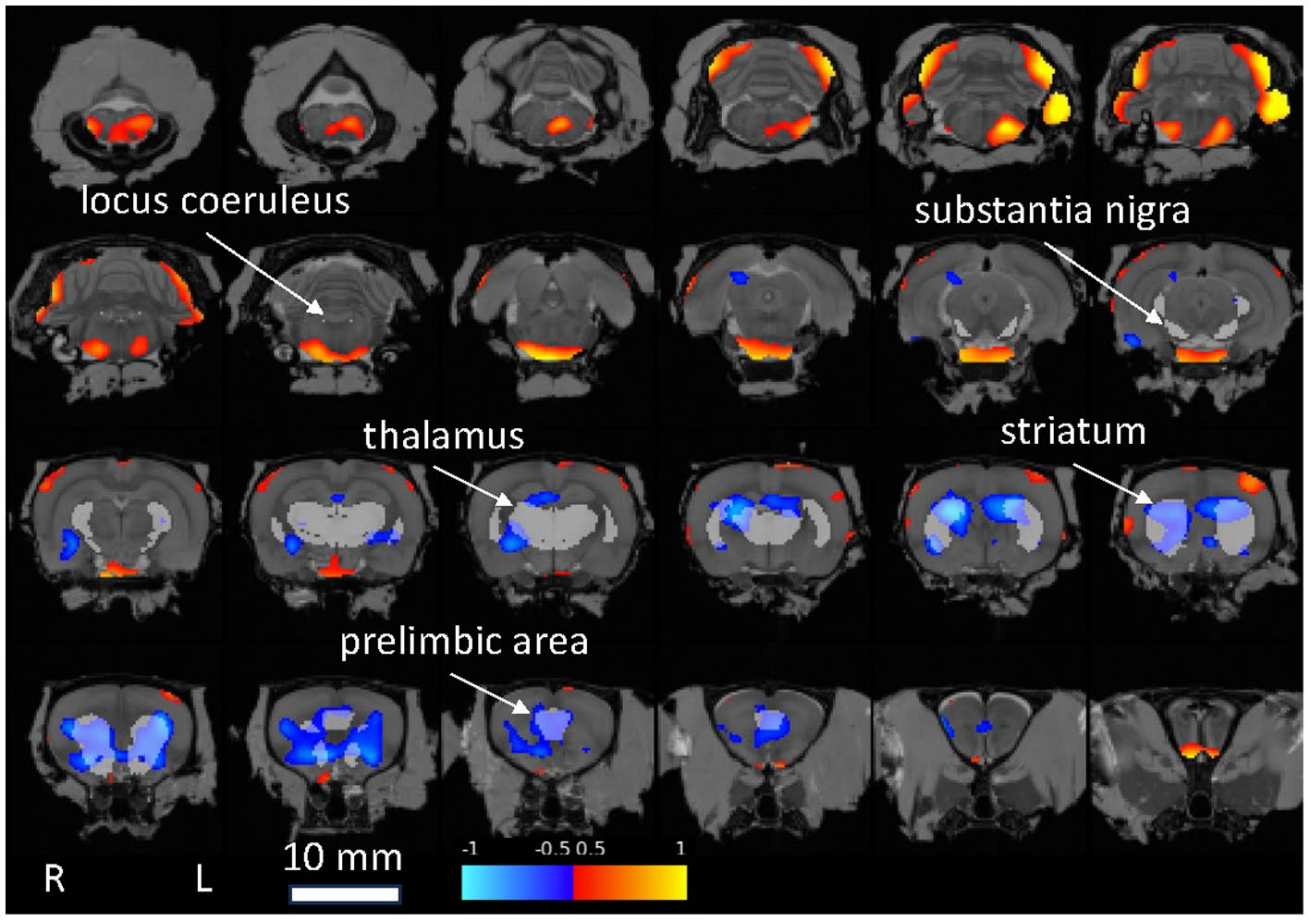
Statistical map showing *Pink1−/−* whole-brain normalized FDG uptake images compared against wildtype images (two-sample *t*-test). Red: *Pink1−/−* > wildtype; blue: *Pink1−/*− < wildtype (0.05 < |t| < 0.10); 1 mm coronal slices overlaid on MRI template image; gray regions of interest indicated by arrows are anatomically defined noradrenergic (locus coeruleus, thalamus, and prelimbic area) and dopaminergic (substantia nigra, striatum, and prelimbic area) structures.
